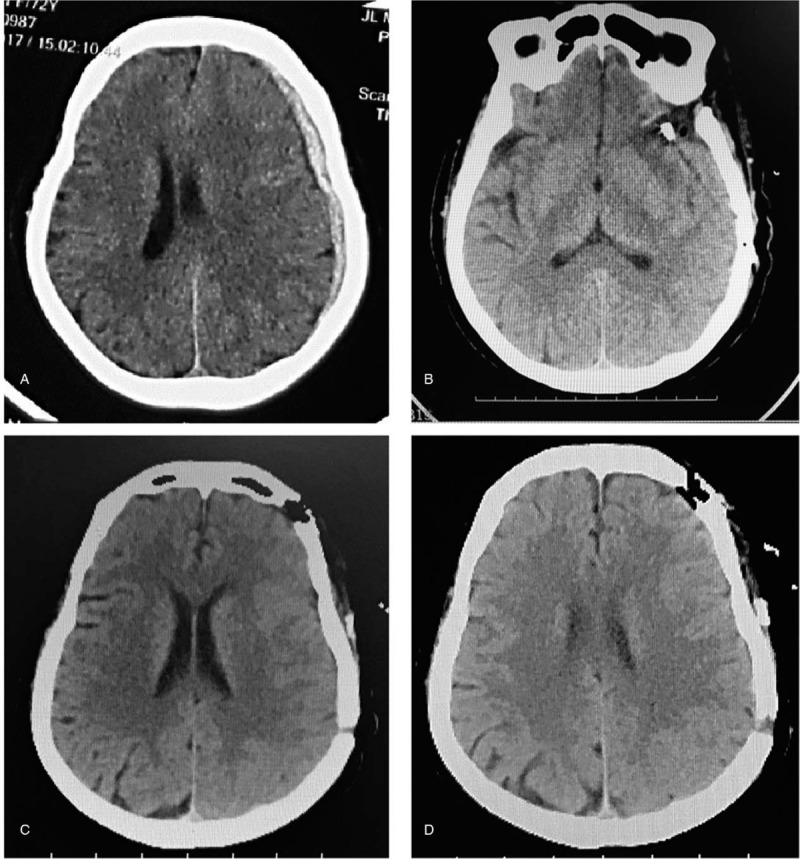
Computed tomography scans taken before surgery (A) and 2 days after surgery (B–D).
